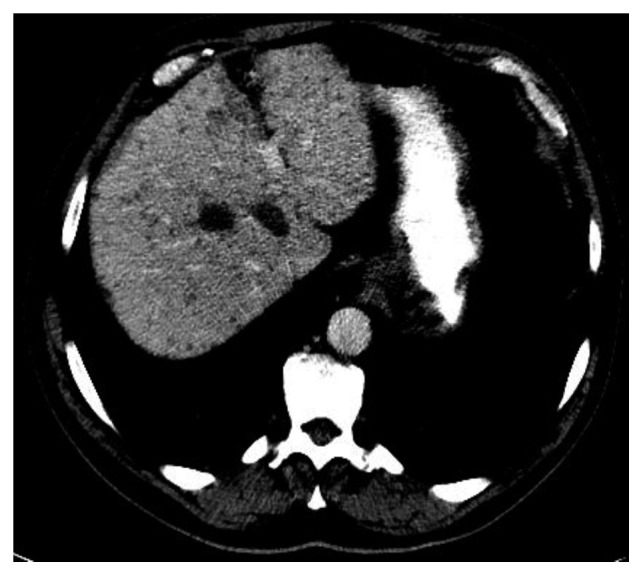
Surveillance CT abdomen/pelvis 3 months after the initial one showing numerous new tiny hepatic lesions.
